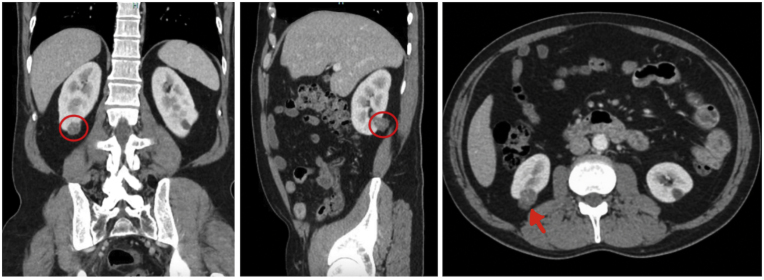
CT views of the right kidney tumor (encircled) with a slight bulge toward the sinus fat.
